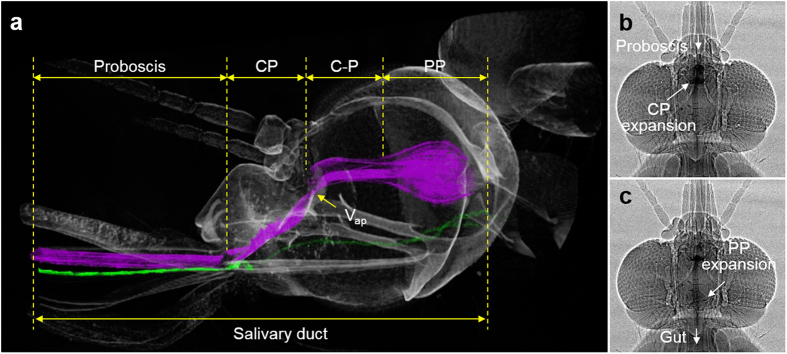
Typical morphology of the heads of a female mosquito (a), and sequential expansion and contraction of the CP (b) and PP (c) pumps. Figures show the functional features of the pump systems of *An. sinensis.* 3D-reconstruction image of the head part and sequential images of the pumps were obtained using X-ray micro-imaging technique.
